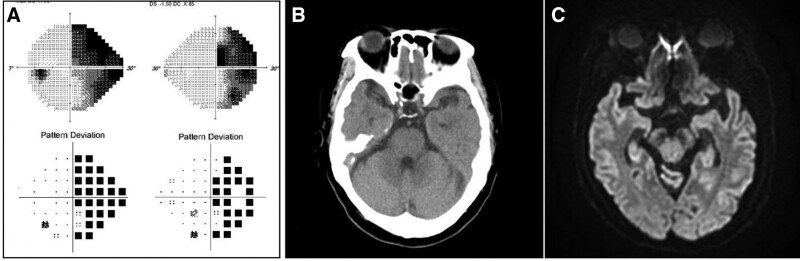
Humphrey visual field (24-2 HVF) testing and brain imaging at the patient’s first visit. (A) Right homonymous hemianopia is noted in the HVF test. (B) No evidence of hemorrhage or mass is seen on brain CT. (C) In brain diffusion MRI, no pathologic lesion is noted in the brain parenchyma. CT = computed tomography, MRI, magnetic resonance imaging.
